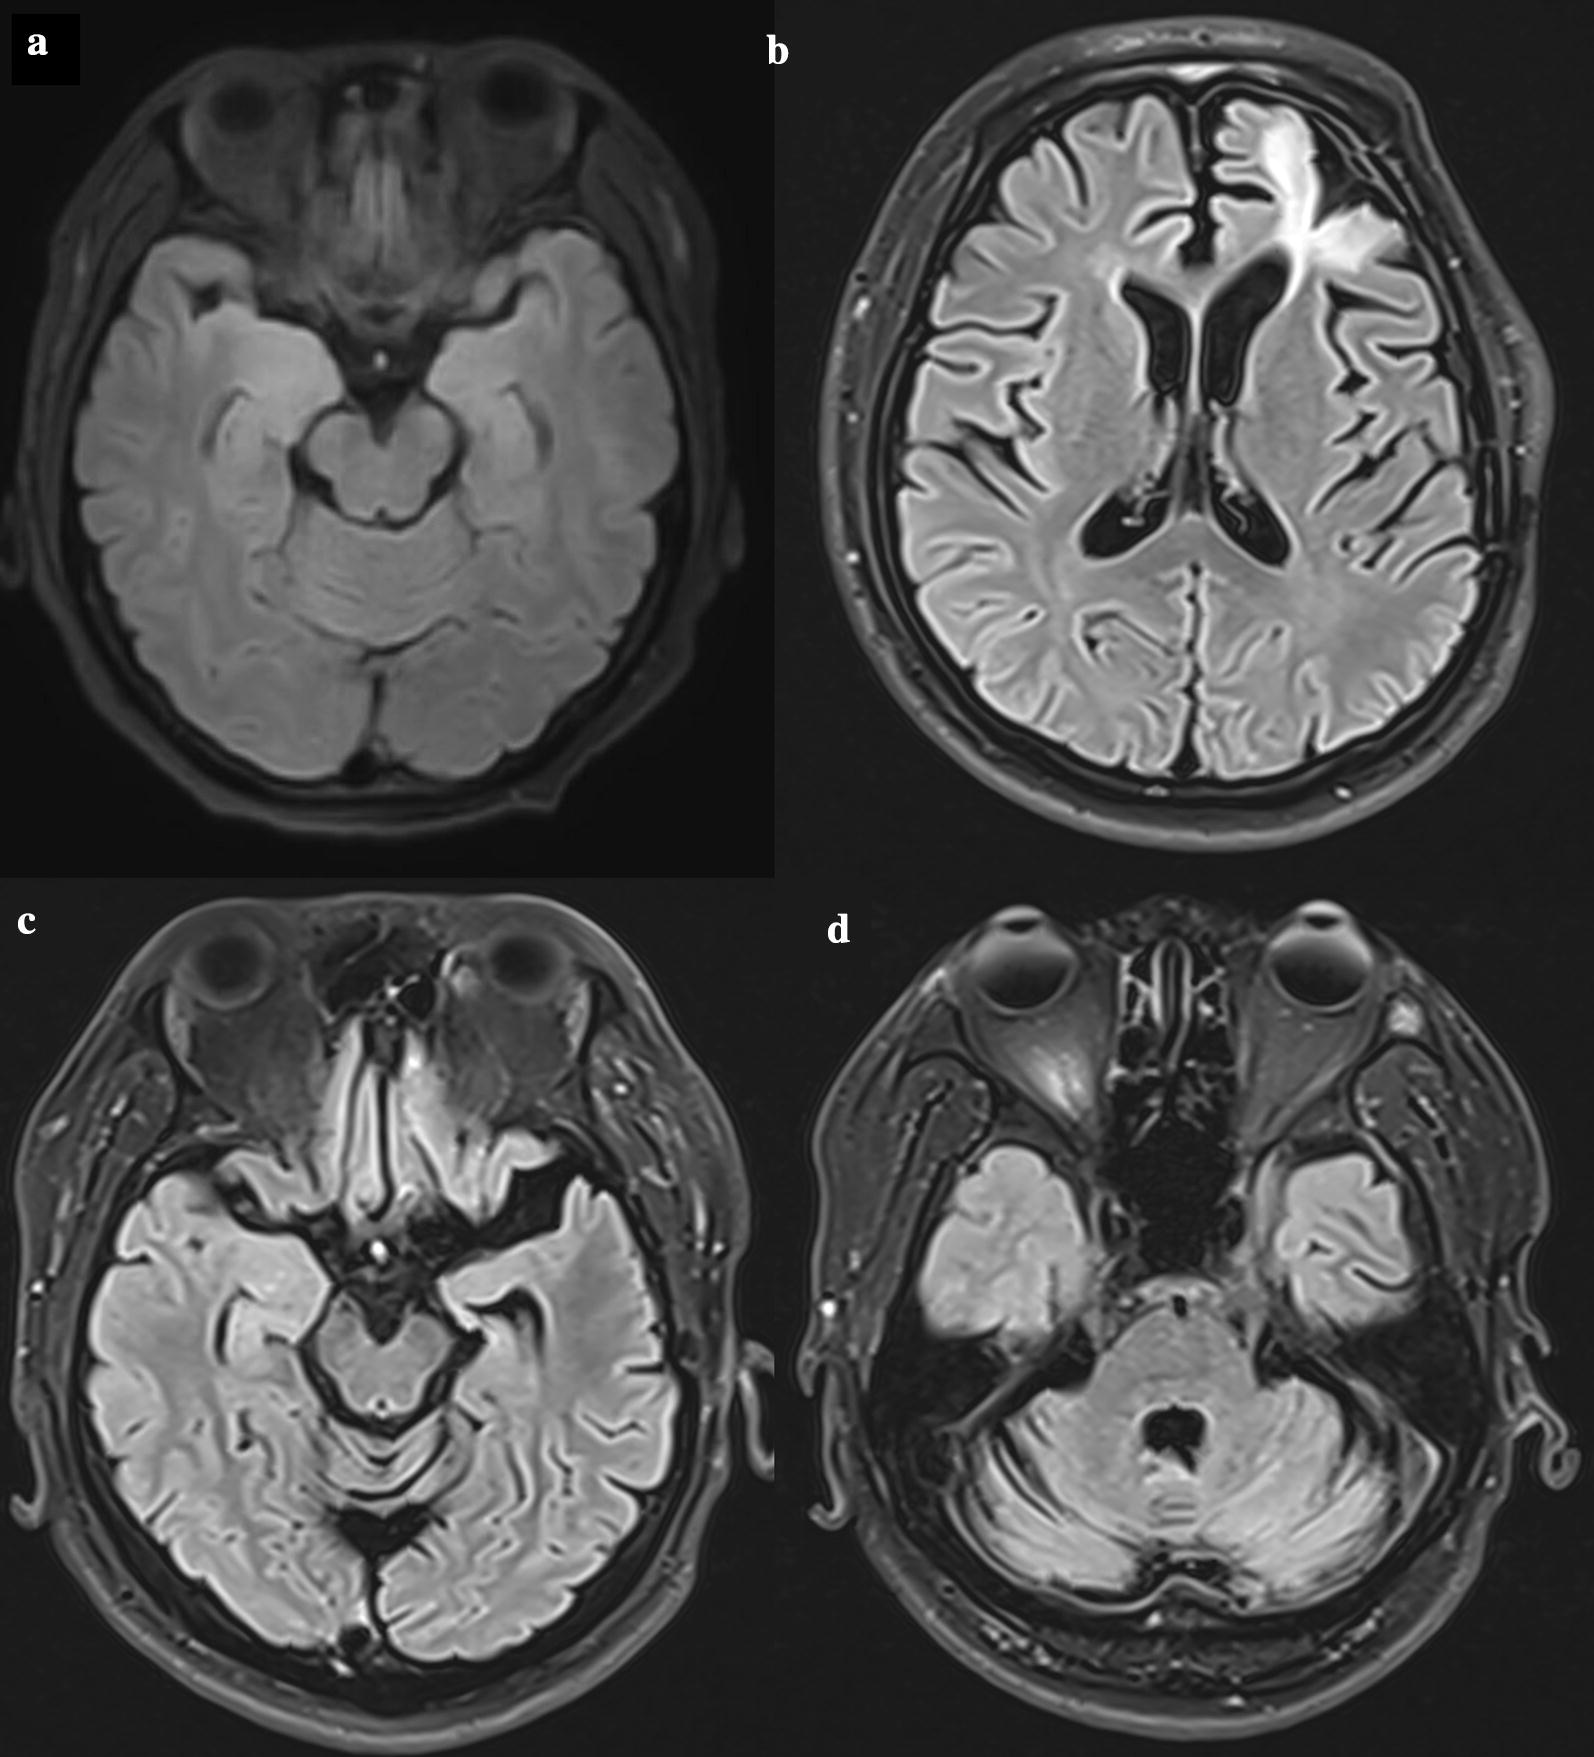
Axial magnetic resonance imaging (MRI) findings of patient 1 and patient 2 in fluid-attenuated inversion recovery (FLAIR) sequence. a Patient 1, a 35-year-old Chinese female, was diagnosed with typical limbic encephalitis, and MRI showed increased FLAIR signals of the right medial temporal lobe, including the amygdala and hippocampus. b–d Patient 2, a 35-year-old male, was characterized by stiff-person syndrome, cerebellar ataxia and intractable epilepsy. MRI indicated postoperative changes in the left frontal lobe, volume reduction in the left temporal lobe and hippocampus, and encephalatrophy, especially in the bilateral cerebellum
